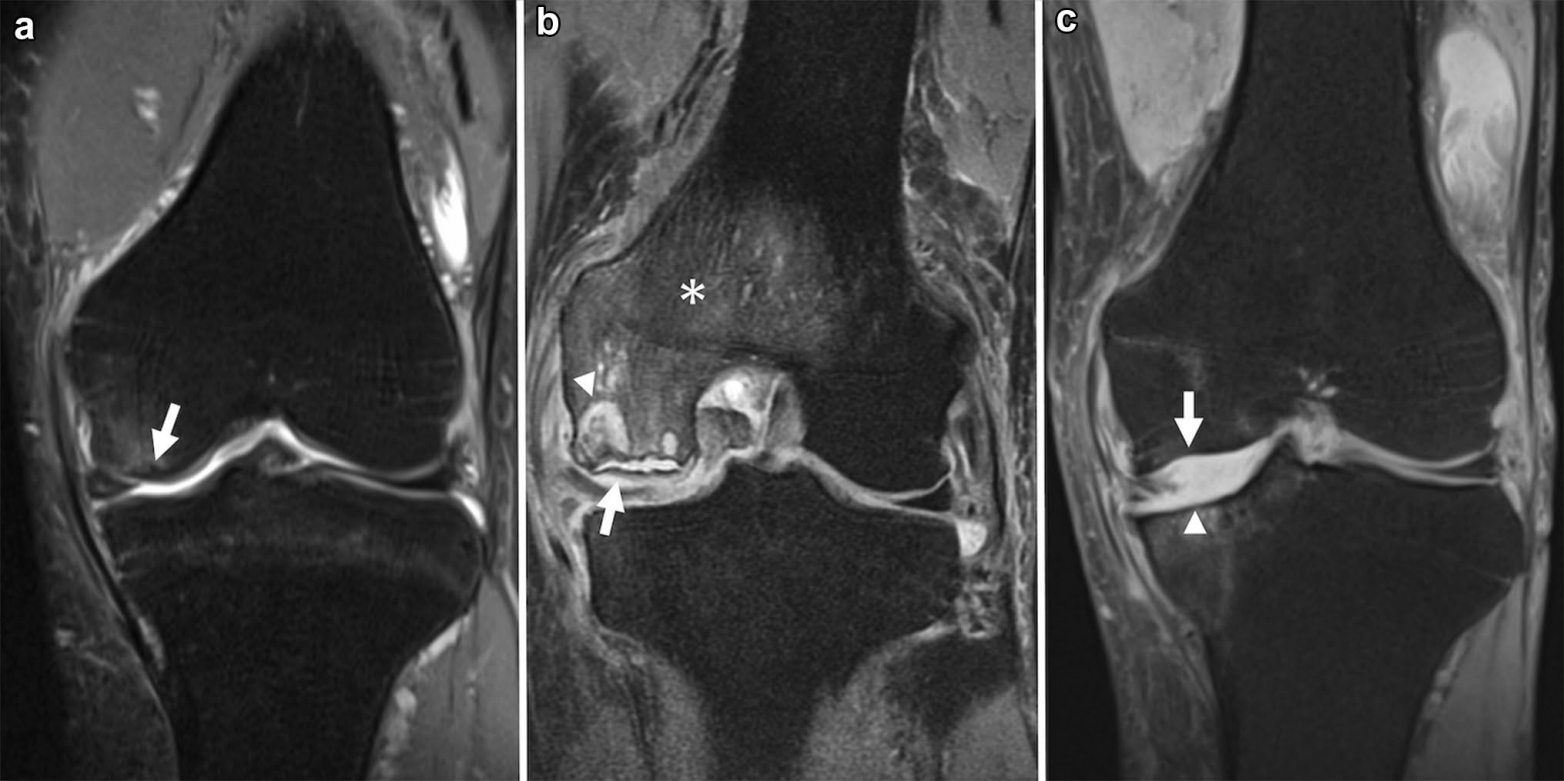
Coronal FS-PDWI of a 71-year-old female patient with SIFK in the medial femoral condyle of the left knee. a The initial MR image shows a subchondral hypointense line (arrow) with subtle bone marrow edema-like signal intensity around the lesion. b An MRI obtained 6 months later shows a fluid-filled cleft under the subchondral plate (arrow) and a large cyst with necrotic bone fragments beneath the lesion (arrowhead). The bone marrow edema-like signal intensity appears to expand over the medial femoral condyle (asterisk). c An MRI obtained 4 years later shows an osteochondral defect of the condyle (arrow). There is also an osteochondral defect on the tibial side (arrowhead) because the patient developed another SIFK in the medial tibial condyle during the disease
